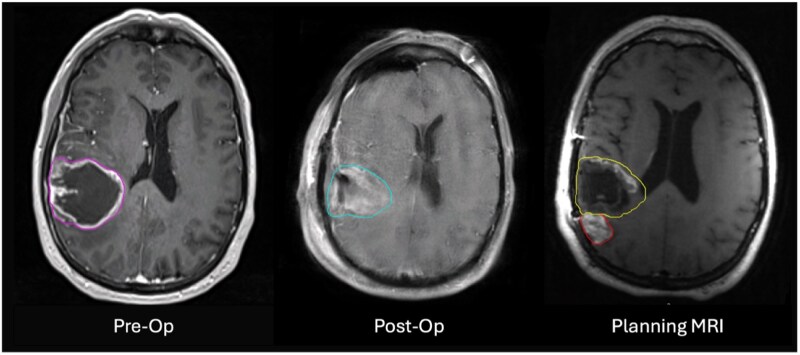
T1 gadolinium enhanced MRIs were used to contour tumor and cavity volumes at 3 time points: pre-operative, post-operative and radiotherapy (RT) planning. Pictured above are the Pre-operative GTV (left), post-operative cavity (center), planning cavity and GTV/rapid early progression (REP) (right). On the planning MRI, the cavity contour is shown superiorly while REP is shown inferiorly. REP here is defined as an increase in enhancing tumor volume between post-operative and pre-RT imaging (≥2 cc). The region of REP represents this increase enhancing tumor. Slices were aligned using anatomical landmarks, slight variations in positions may be due to post-operative changes such as mass effect.
